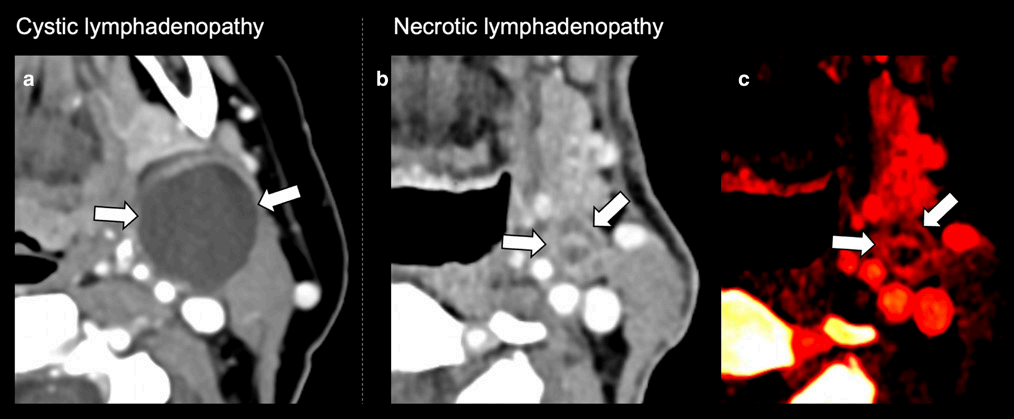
Differentiation of cystic and necrotic cervical lymphadenopathy using cross-sectional imaging. Post- contrast single energy (A) and dual-energy (B, C) CT images demonstrating nodal features described by Goldenberg et al.^
